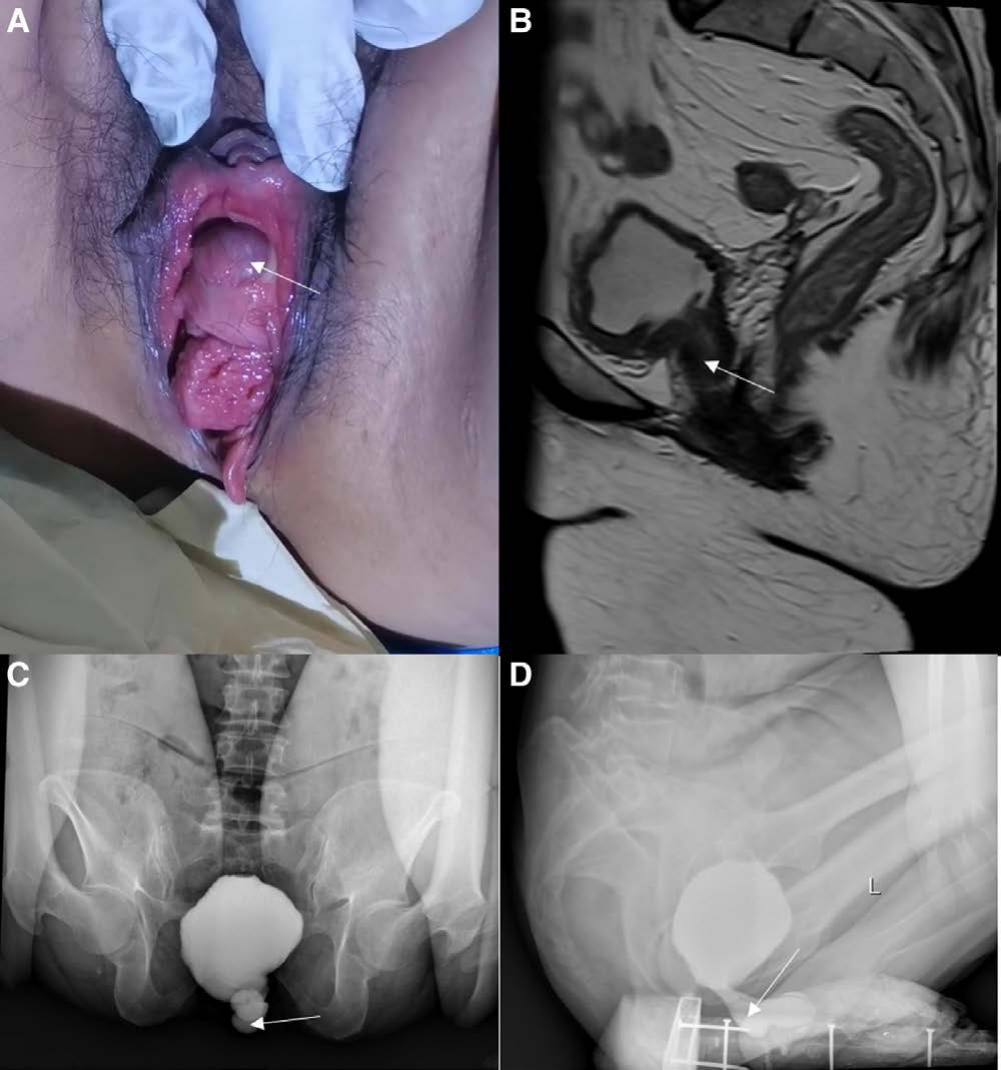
(A). Cystic protrusion of the posterior urethral wall. (B–D) The radiological manifestations of bladder diverticulum on MRI and VCUG. VCUG = voiding cystourethrography.
